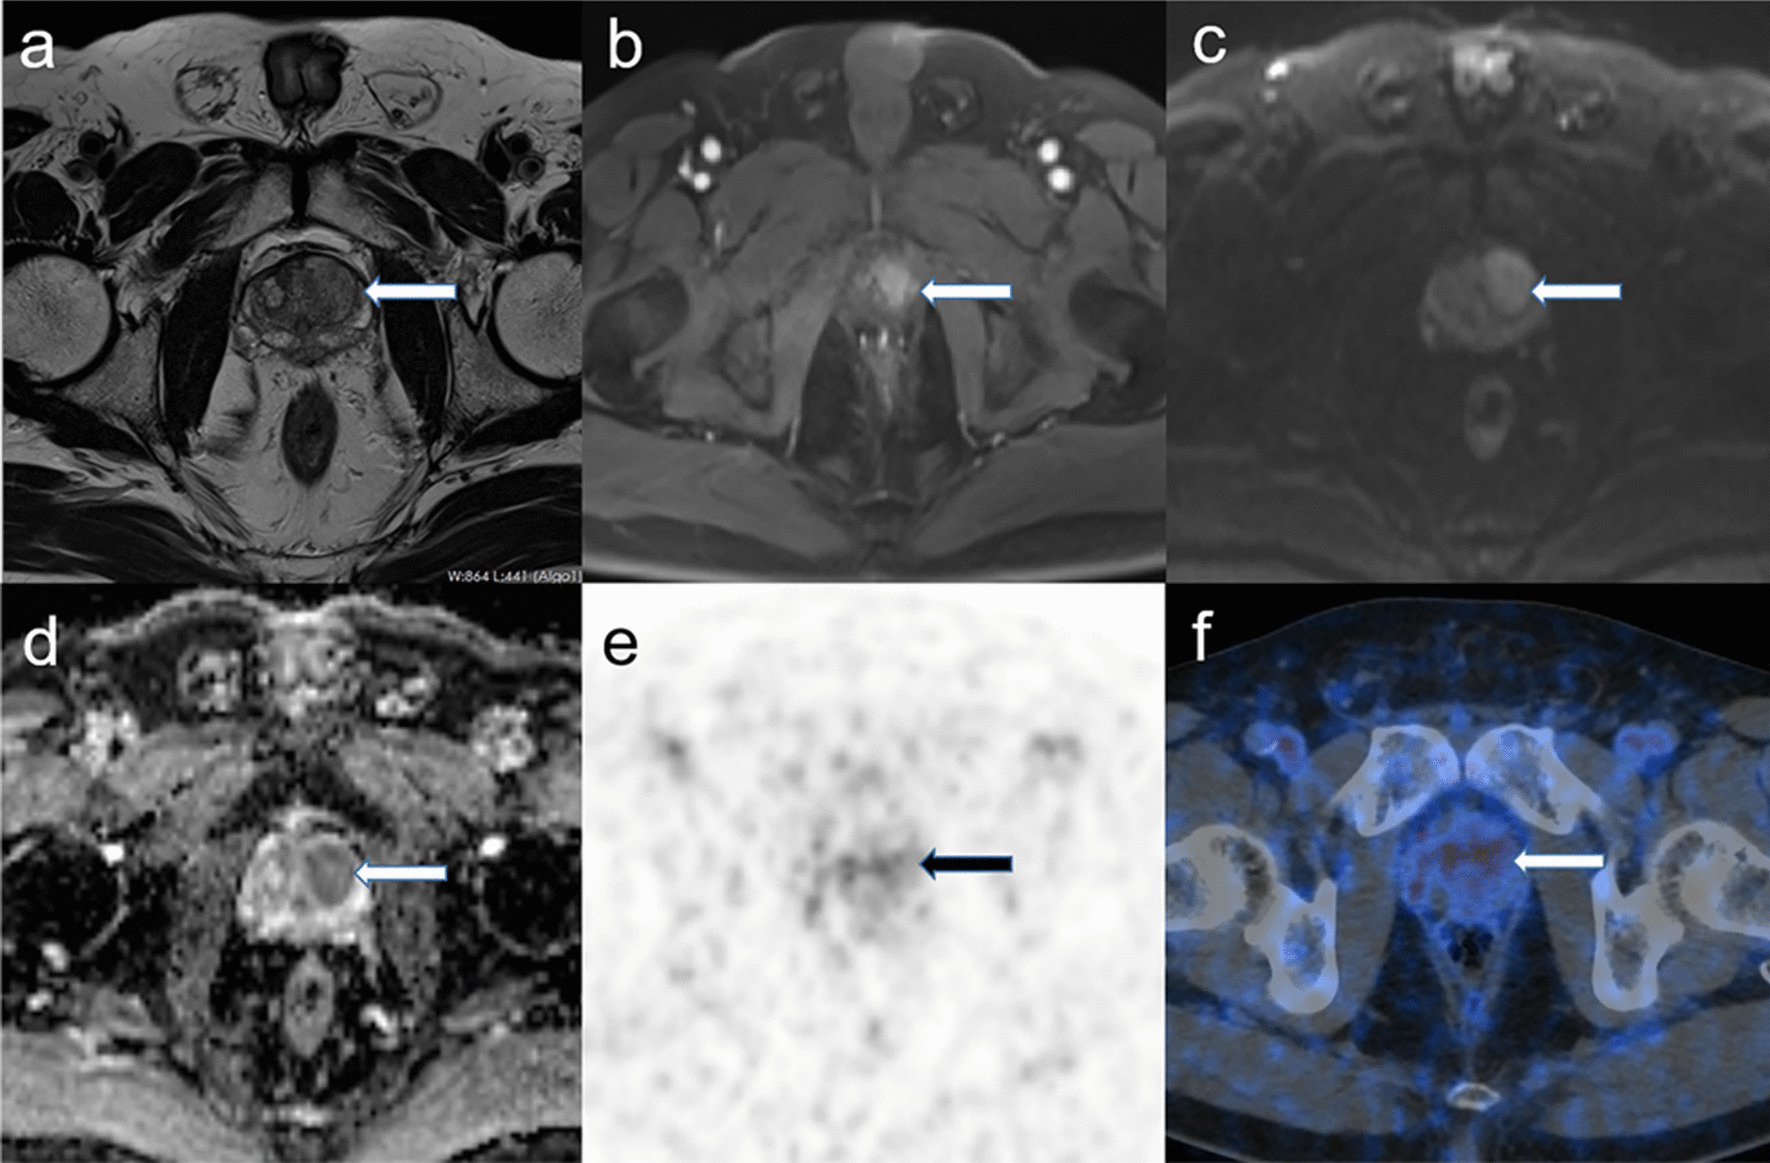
Example of an inconsistent evaluation using mpMRI (+) and ^68^Ga-PSMA PET/CT (−) in low- and intermediate-risk PCa. A 59-year-old patient whose serum PSA level was 8.0 ng/ml, and GS was 3 + 3. There is a lesion in the left transition zone of the prostate gland in the pelvic MRI scan (a T2w, arrow; b contrast-enhanced T1w sequence, arrow), and a diffusion restriction (c b 1500 DWI, arrow; d corresponding ADC map, arrow) makes the presence of a large PCa very likely. In the ^68^Ga-PSMA PET/CT there is no strong tracer uptake highly likely to not be diagnosed (arrow in e and f). a–d MRI; e–f
